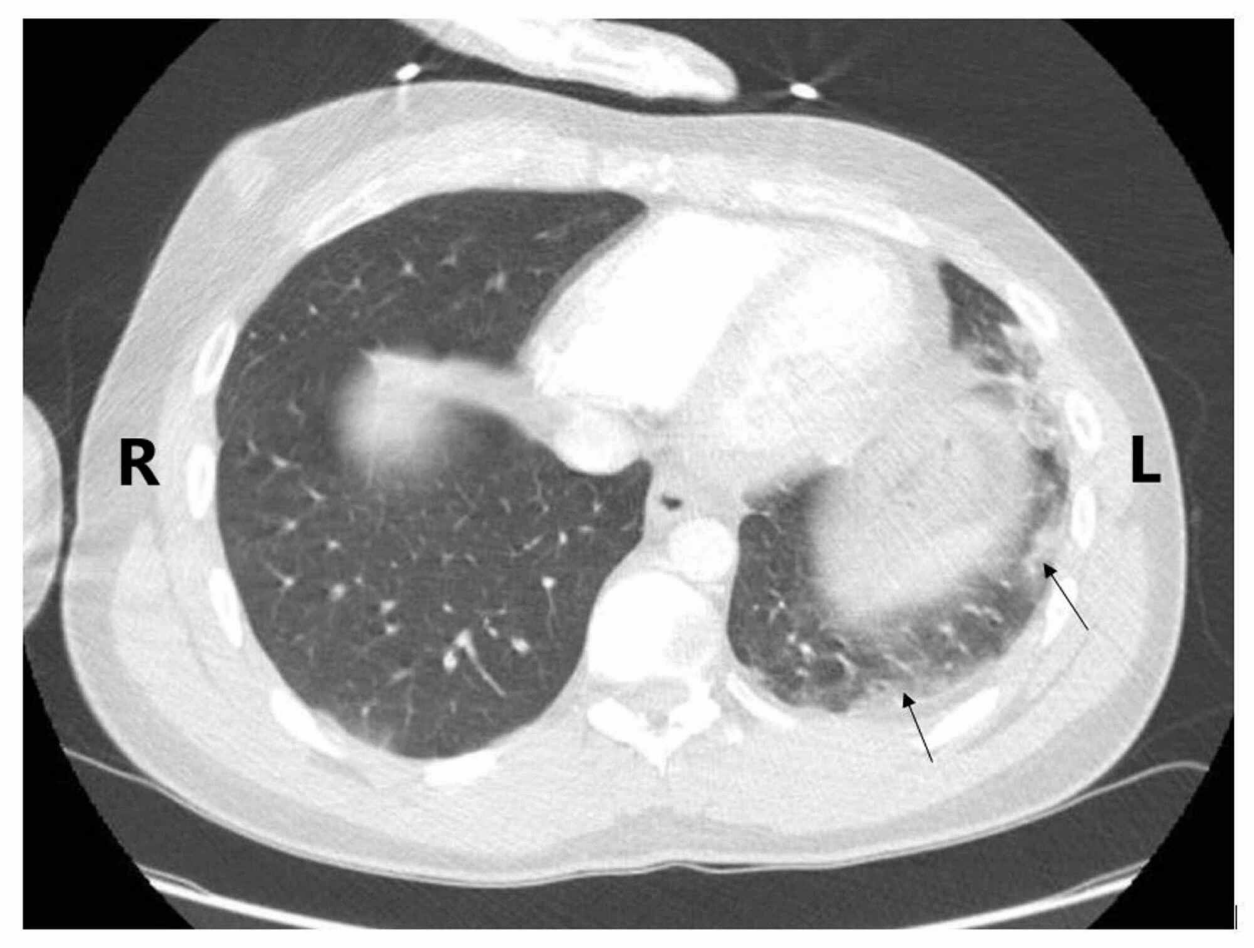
CTPA showing bilateral basal infiltrates, particularly on the left side in this section (see arrows), highly suggestive of COVID-19. CTPA, computed tomography pulmonary angiogram
